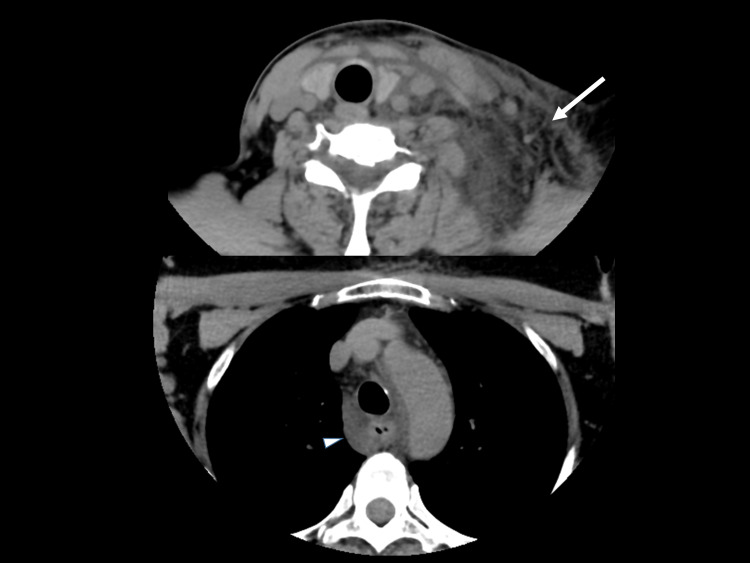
Trunk computed tomography (CT) on arrival CT showed left-side-limited edema (arrow) in the subcutaneous area and surrounding esophagus and bronchus (arrow head).
